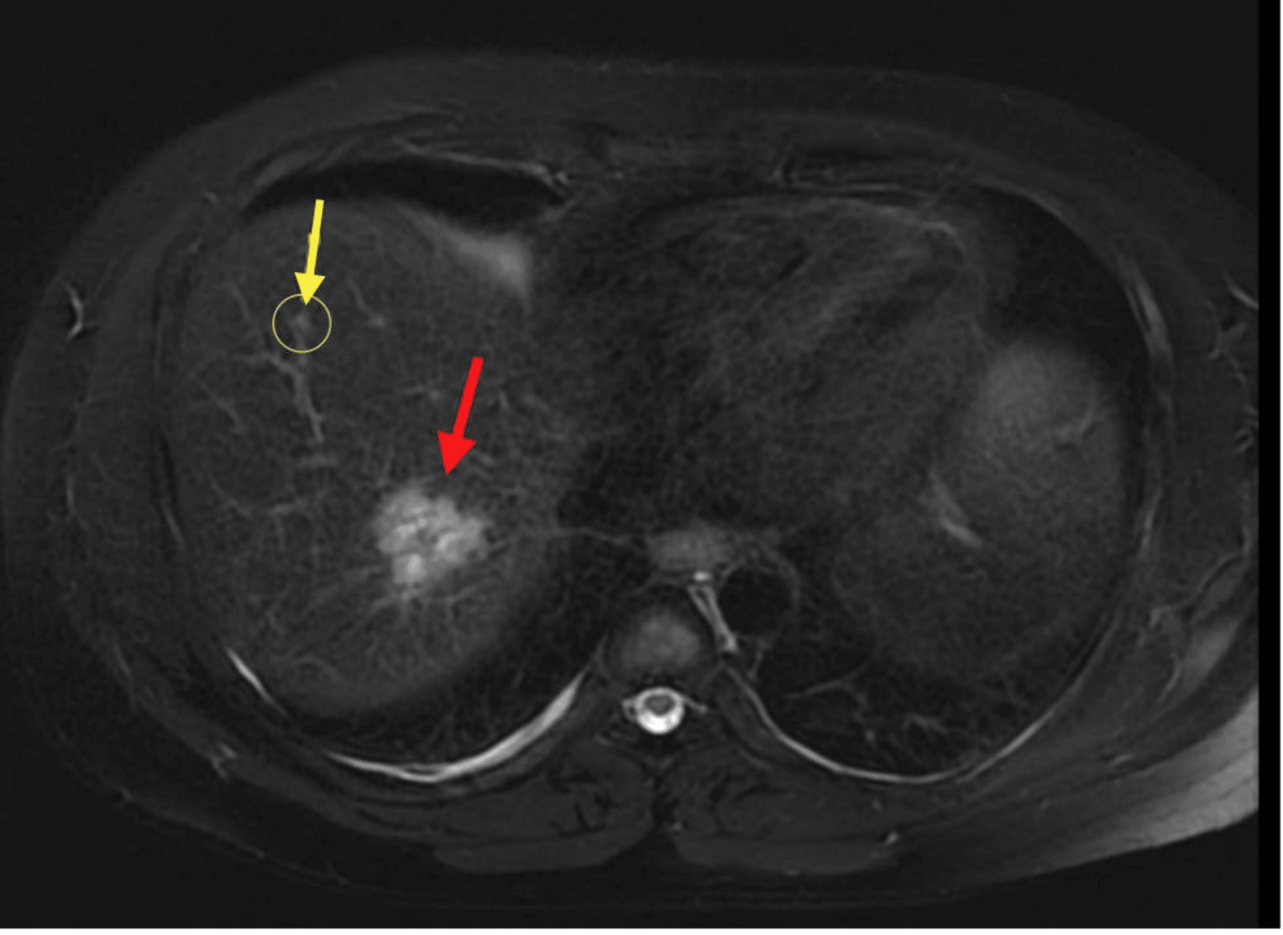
Abdominal MRI showing multiple liver lesions: yellow arrow showing a lesion in segment 8 and red arrow showing a lesion in segment 7 MRI: magnetic resonance imaging
